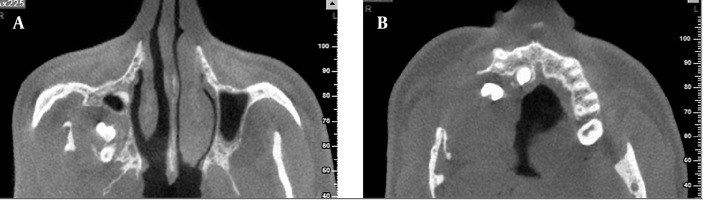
CBCT findings. A and B, Axial CBCT views indicate impacted teeth, mass effect on the posterior and lateral walls of the right maxillary sinus toward the antral cavity and involvement of the mandibular ramus.
